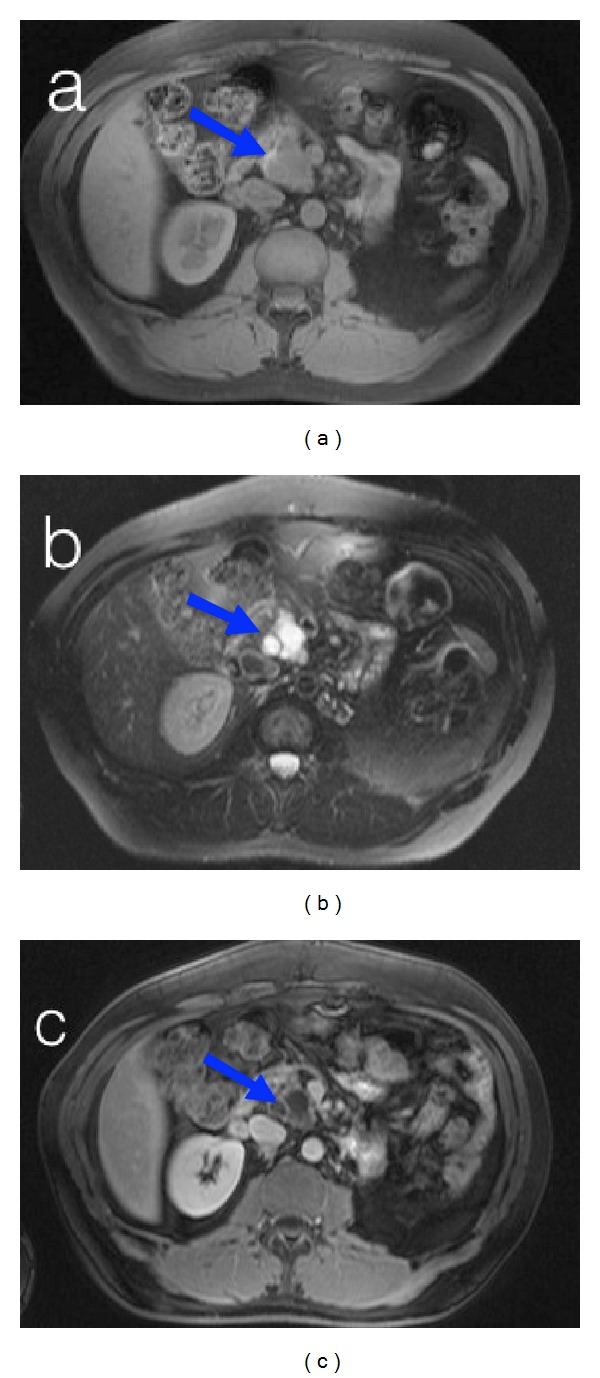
The scans show a multichambered lesion, 3.3 × 2.5 cm in size, localized in the head and in the uncinate process of the pancreas, hypointense in T1-weighted image (a) and hyperintense in T2-weighted image (b). After the intravenous contrast medium administration (c), the enhancement of the capsule and internal septa was shown. (a) Precontrast axial T1-WI FAT SAT. (b) Precontrast axial T2-WI FAT SAT. (c) Postcontrast axial T1 WI. The blue arrow indicates the lesion.
